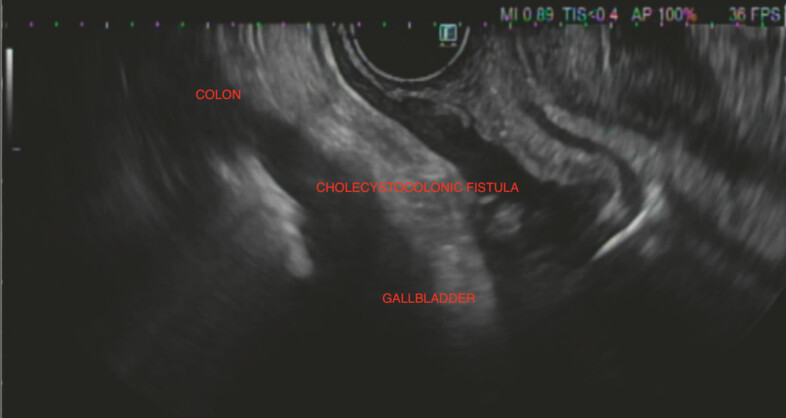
EUS demonstrating gallbladder with intraluminal air and communication with the colon. EUS, endoscopic ultrasound.
